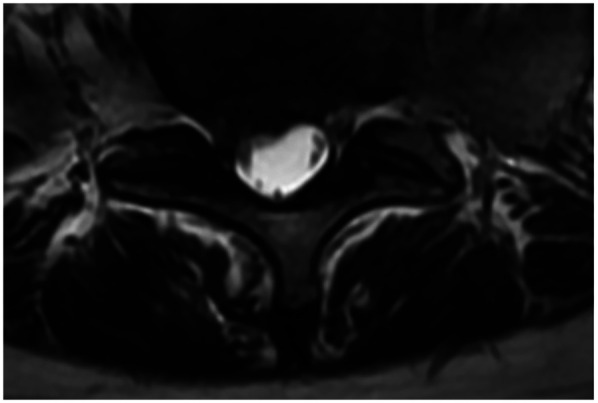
Preoperative and postoperative axial T2-weighted MRI scans. (A) Preoperative image showing a herniated intervertebral disc (red arrow) compressing the nerve root. (B) Postoperative follow-up demonstrating adequate decompression, with the nerve root clearly released and no residual compression at the surgical level.
